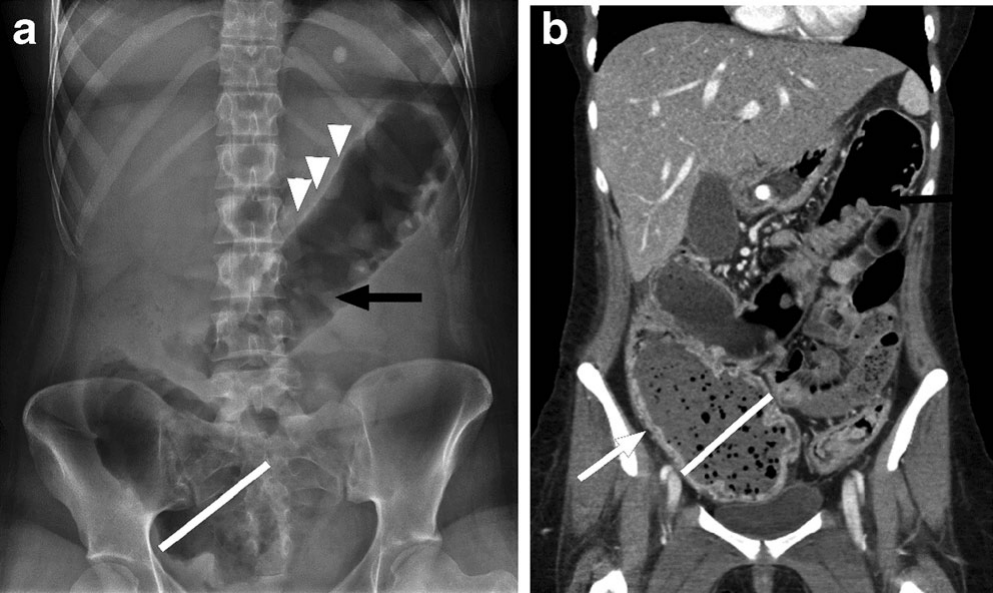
a Abdominal radiograph of a 20-year-old female with SCI and abdominal pain shows a dilated ascending and transverse colon (*white line*) with loss of colonic haustrations (*white arrowheads*) and pseudo-poly formation of the colonic mucosa (*black arrow*), concerning for toxic mega colon. b The corresponding coronal CT images confirm radiographic findings of bowel dilatation (*white line*), bowel wall thickening and oedema (*white arrow*) and mucosal pseudo-polyps (*black arrow*) typically associated with toxic megacolon
